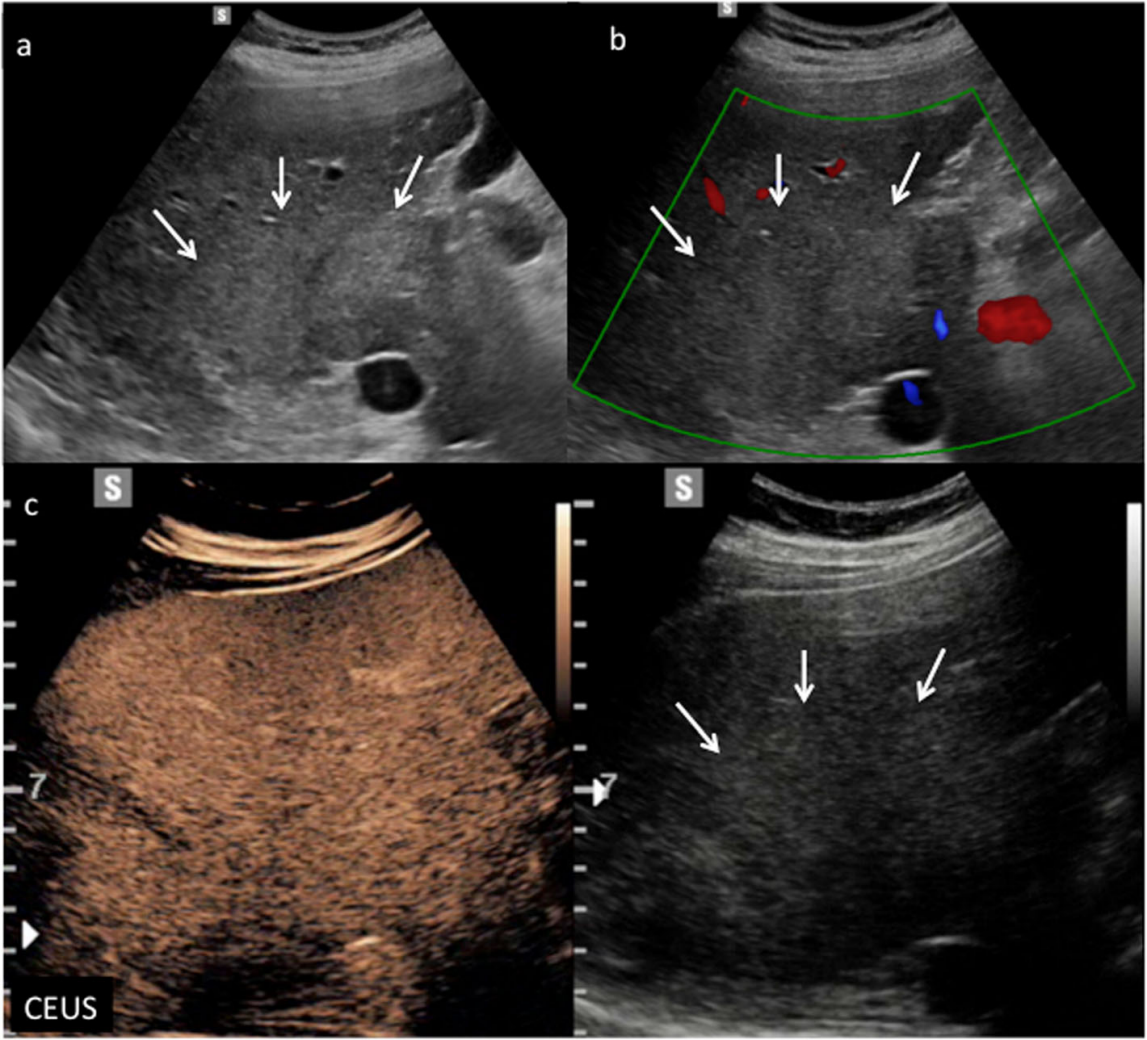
CEUS LI-RADS 2 (probably benign). Non mass-like area in a 57-year-old man with virus C-related cirrhosis. Baseline US image (a) shows a slightly hyperechoic area with indistinct margins sized 7 cm in the segment V–VI (arrows). The area does not show any vascular signal at color-Doppler (b) (arrows). At CEUS (c), it appears constantly isoechoic with respect to the surrounding liver parenchyma throughout the vascular study (arrows)
